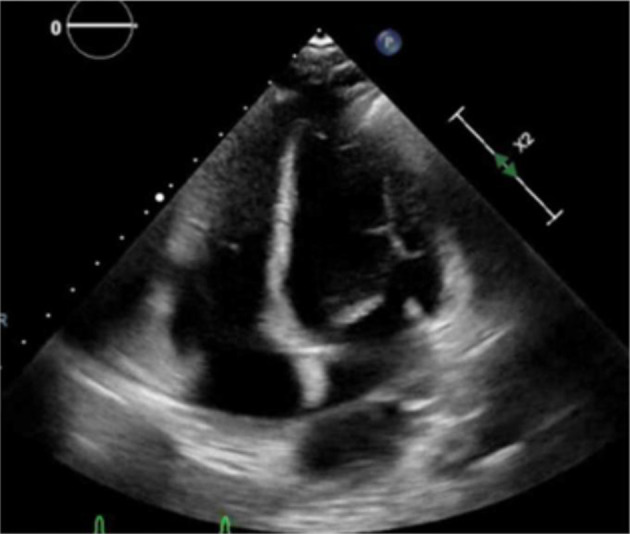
Complete transthoracic echocardiography showing ejection fraction of 55–60% with no significant valvular abnormalities.
